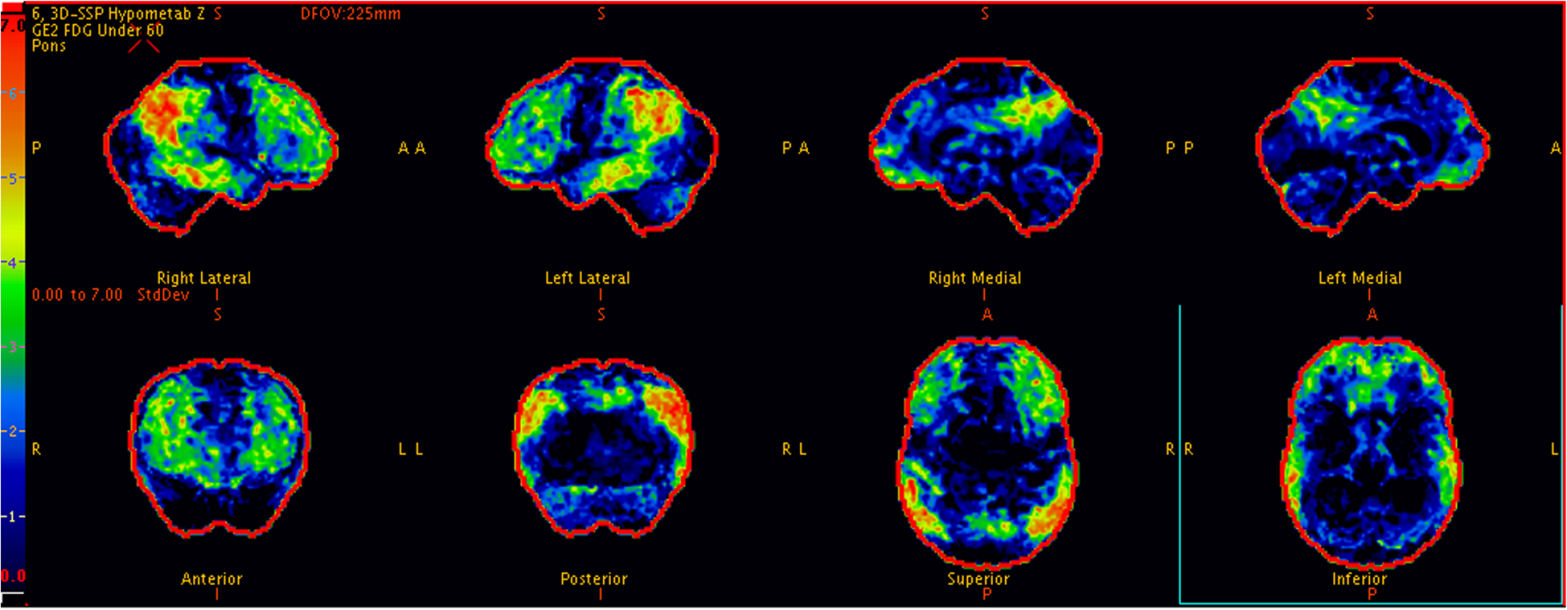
In contrast to Fig. 2, 3d Stereotactic Surface Projection of FDG PET with typical findings of Alzheimer’s disease showing hypometabolism of the bilateral inferolateral temporal lobes, bilateral precuneus, posterior cingulate and angular gyri (parietal lobes). There is relative sparing of the occipital lobes and involvement of the posterior cingulate gyri
